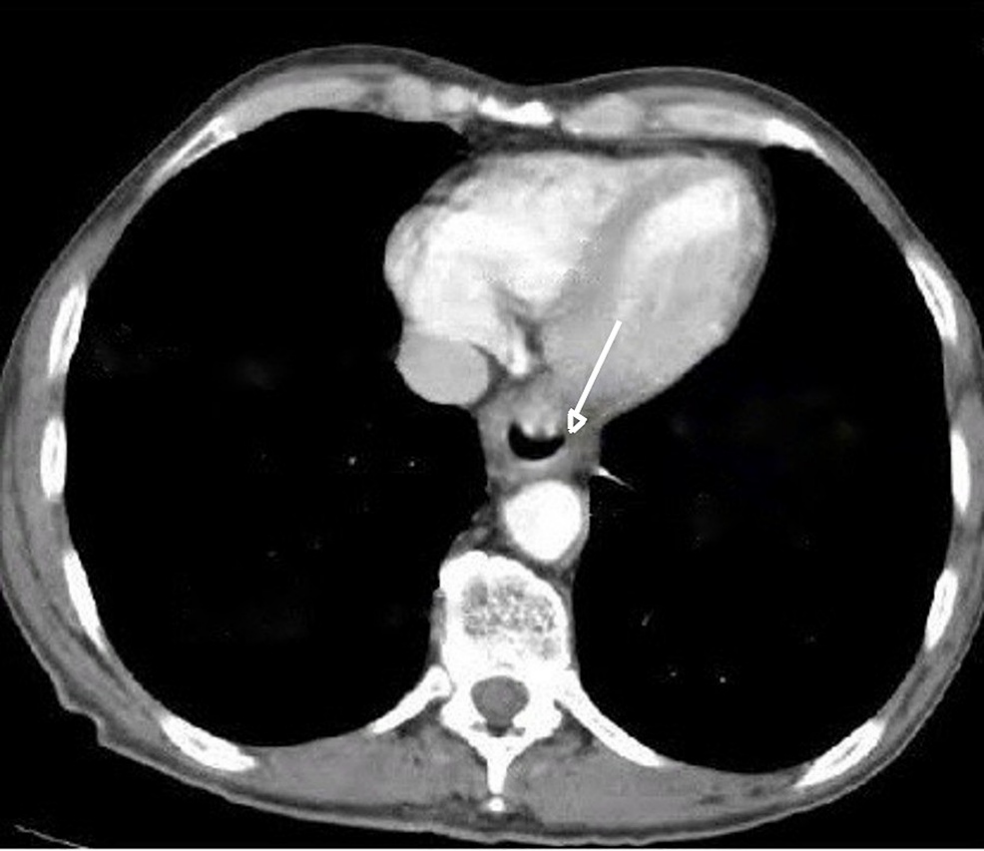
CT image showing a thickening of the wall of the lower esophagus
